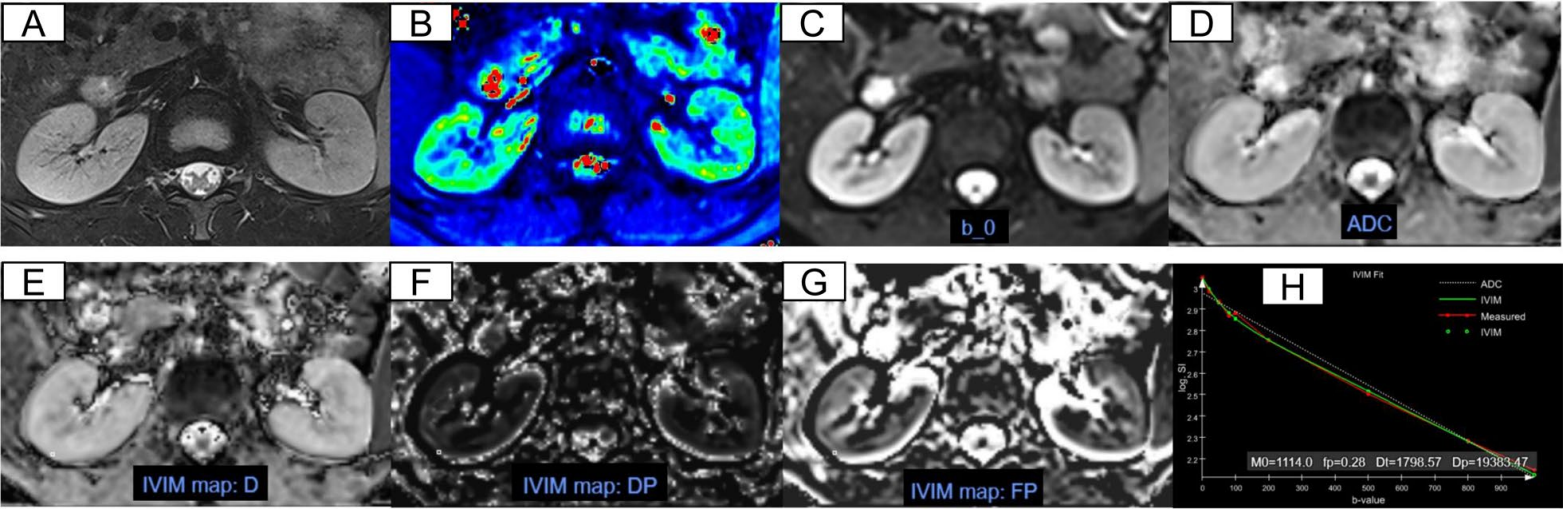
Respective MRI images of healthy volunteers (12 years old, male). A–H axial T2-weighted images, T2* mapping, b0 map, ADC, *D*_*t*_, *D*_*p*_, *f*_*p*_, IVIM fit, respectively
